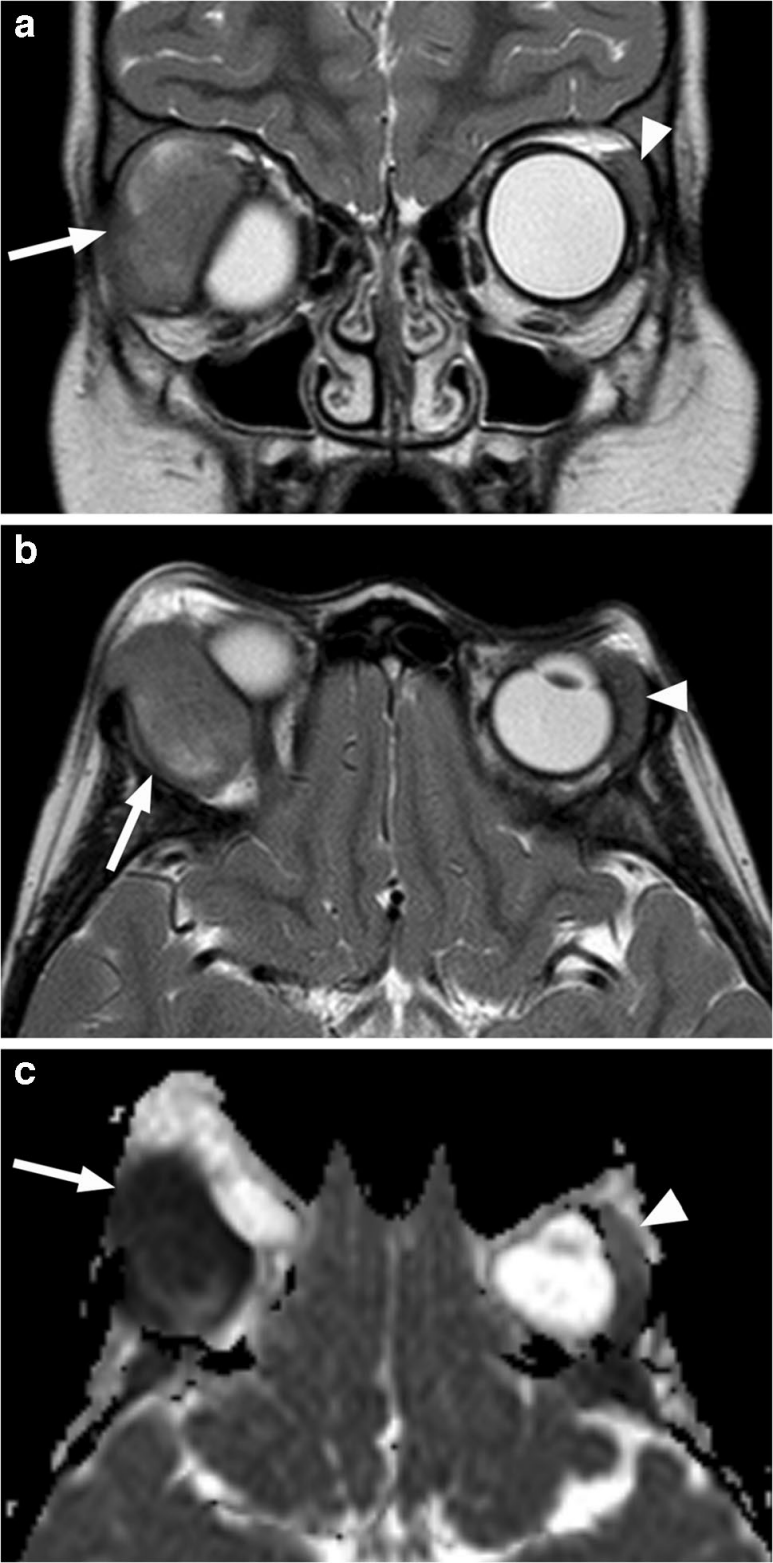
Coronal (a) and axial (b) T2-weighted images as well as axial ADC map (c) demonstrate an extraconal slightly heterogeneous mass (arrows) in the superolateral right orbit, likely arising from the lacrimal gland, with compression of the globe. Compared to the normal gland on the left (arrowheads), the lesion shows slightly increased T2 signal and decreased, very low ADC values (hypointense to the brain). The imaging appearances in this patient with acute lymphoblastic leukemia (ALL) are typical for orbital lymphoma, reflecting the similarity of these two mass lesions
